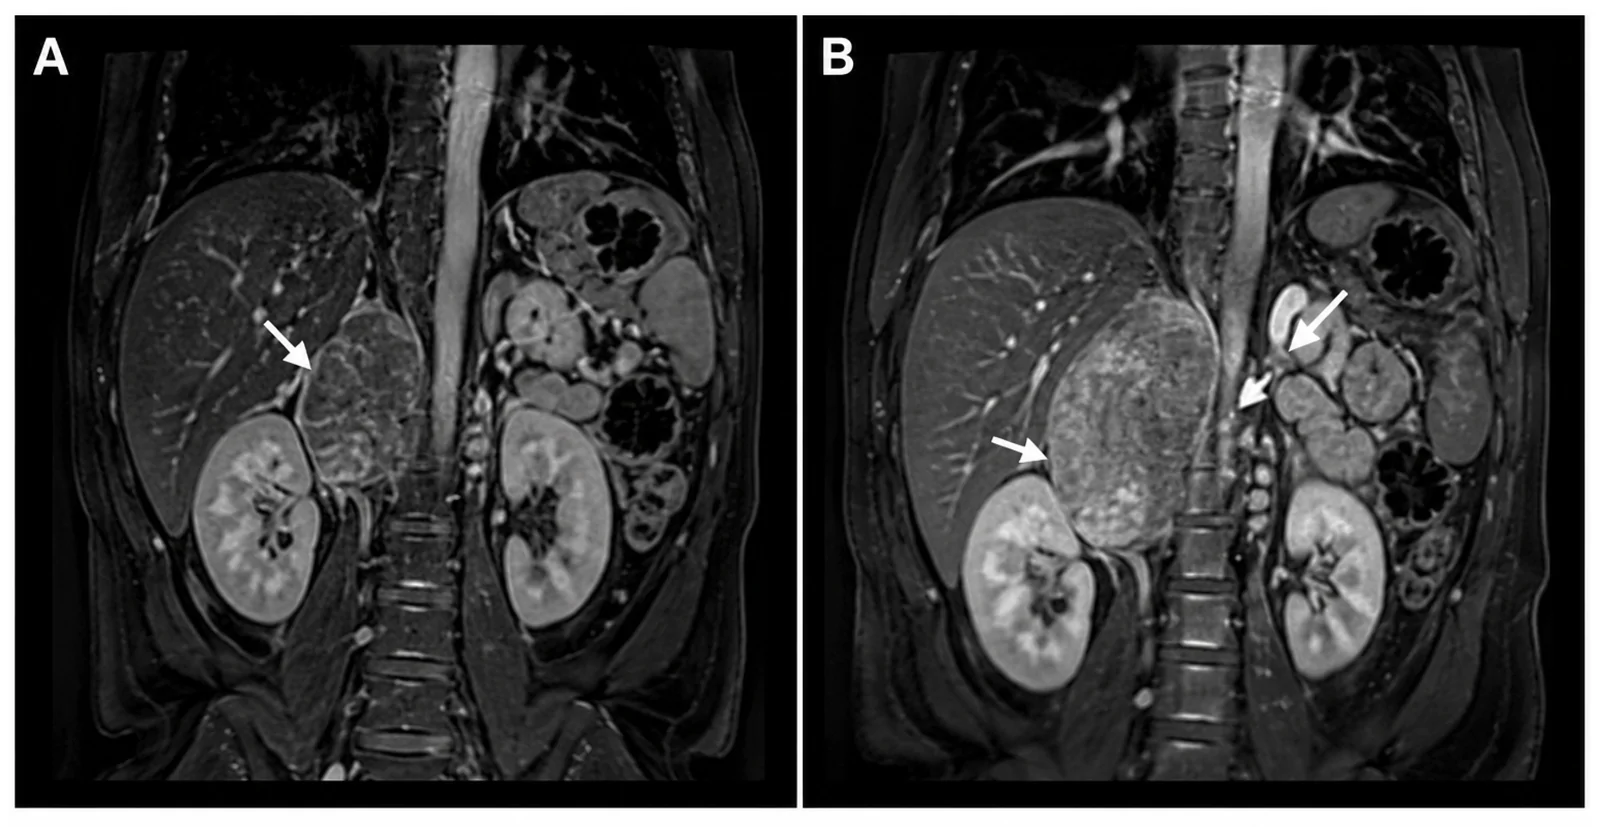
Follow-up contrast-enhanced MRI. (A) Right-sided retroperitoneal/paraspinal tumor in the adrenal region (arrow). (B) Interval tumor enlargement with regional lymphadenopathy (arrows), consistent with disease progression.
